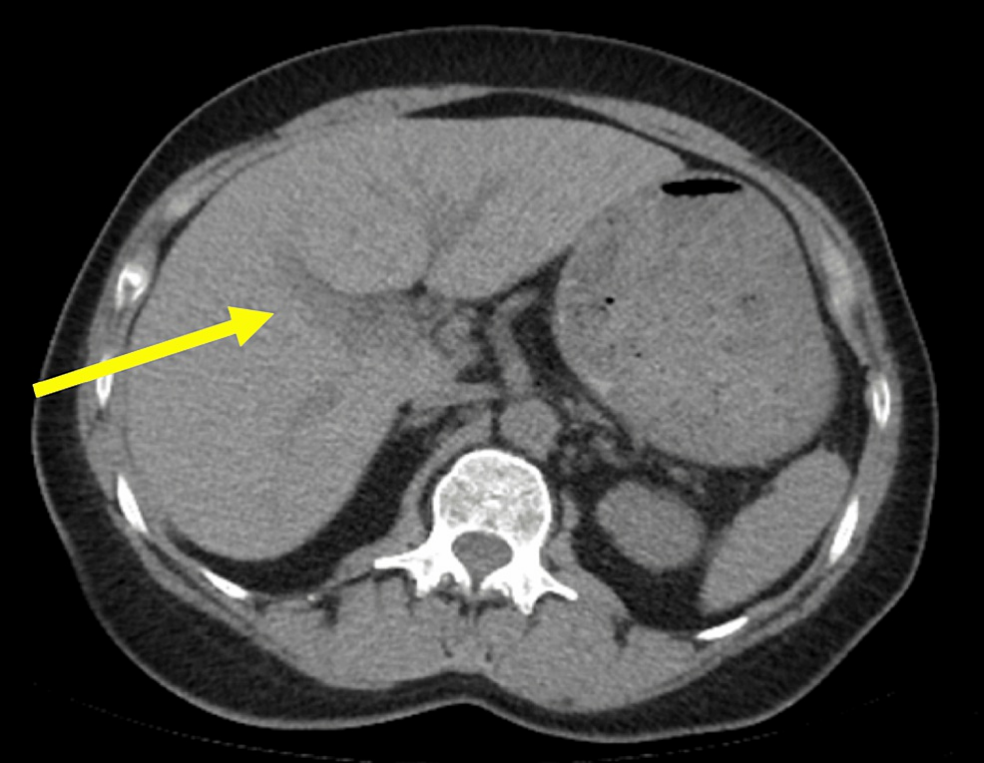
Non-contrast CT scan of the abdomen and pelvis with a transverse view showing subtle periportal edema (yellow arrow). CT: computed tomography.
